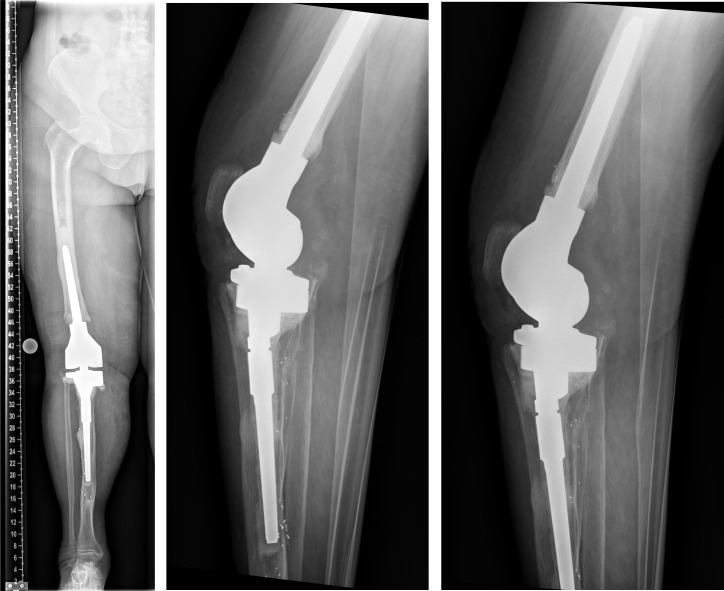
Postoperative X-ray of the right knee joint (in 2016) showing total knee and distal femoral replacement
